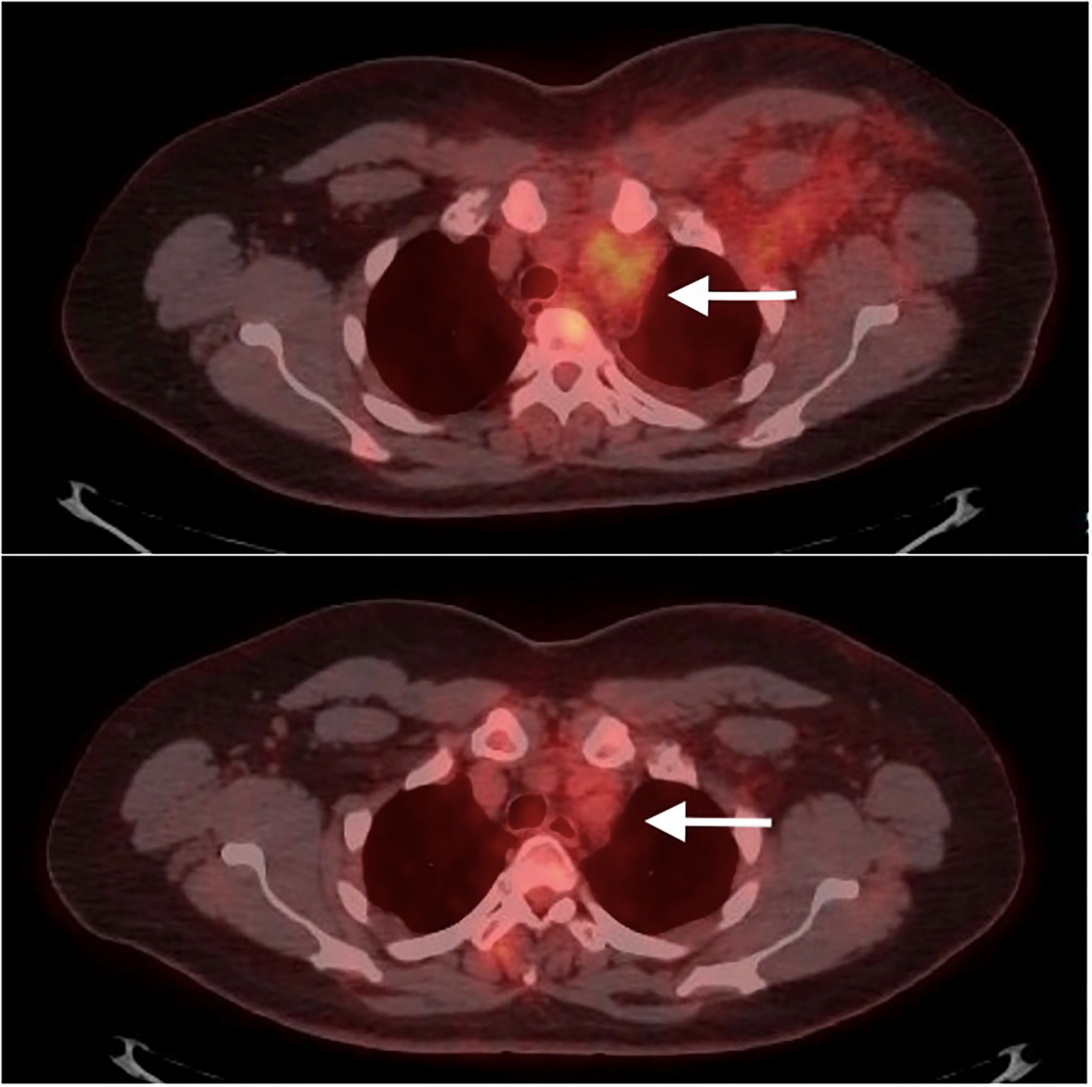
The mediastinal positive PET-CT scan mediastinal mass (above) disappeared after 46 days (below), at the resolution of the SARS-CoV-2.
